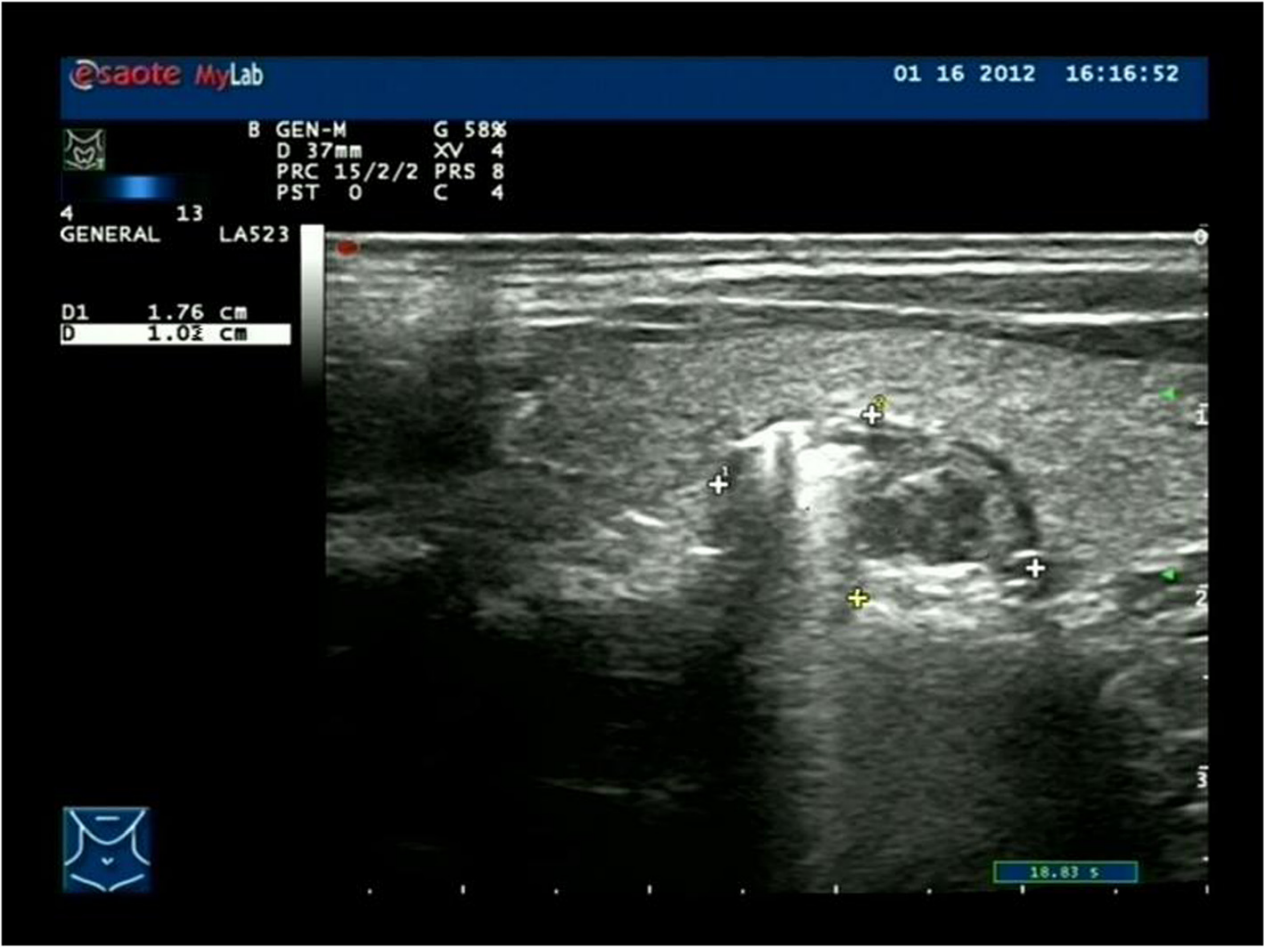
Ultrasound examination showed a solitary, irregular, hypoechoic of mass with post acoustic shadow. Its border was clear and smooth with a max diameter 1.8 cm.
